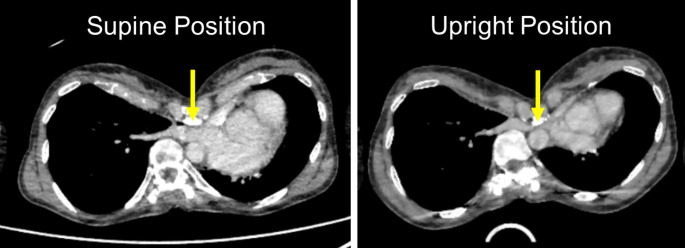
Horizontal CT images at the level of maximal chest depression in a 49-year-old patient with pectus excavatum presenting with upright-worsened dyspnea. Images were obtained during deep inspiration in the supine (left) and upright (right) positions. The right inferior pulmonary vein (yellow arrow) is markedly compressed in the upright position (right)
